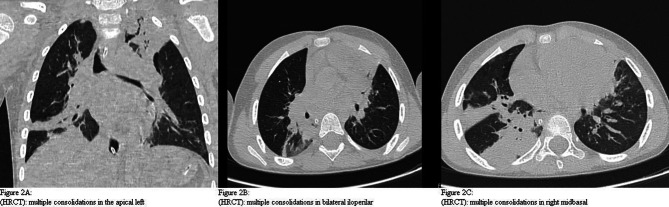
Chest HRCT. (A) Multiple consolidations in the apical left. (B) Multiple consolidations in bilateral iloperilar. (C) Multiple consolidations in right midbasal
